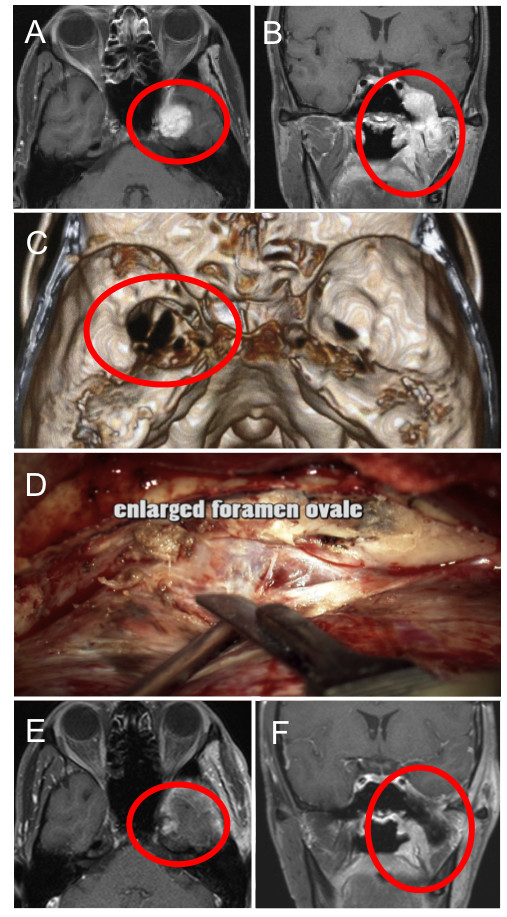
Pre-, Intra-, and Post-operative findings of the present case. A and B, Gadolinium-enhanced T1-weighted magnetic resonance images revealing a mass in the infra-temporal and middle cranial fossa (circle). C, Three-dimensional bone image computed tomography scan demonstrating widening of the foramen ovale (circle). D, Intra-operative finding showing the tumor and enlarged foramen ovale. E and F, Postoperative gadolinium-enhanced T1-weighted magnetic resonance images manifesting removal of the tumor (circle).
